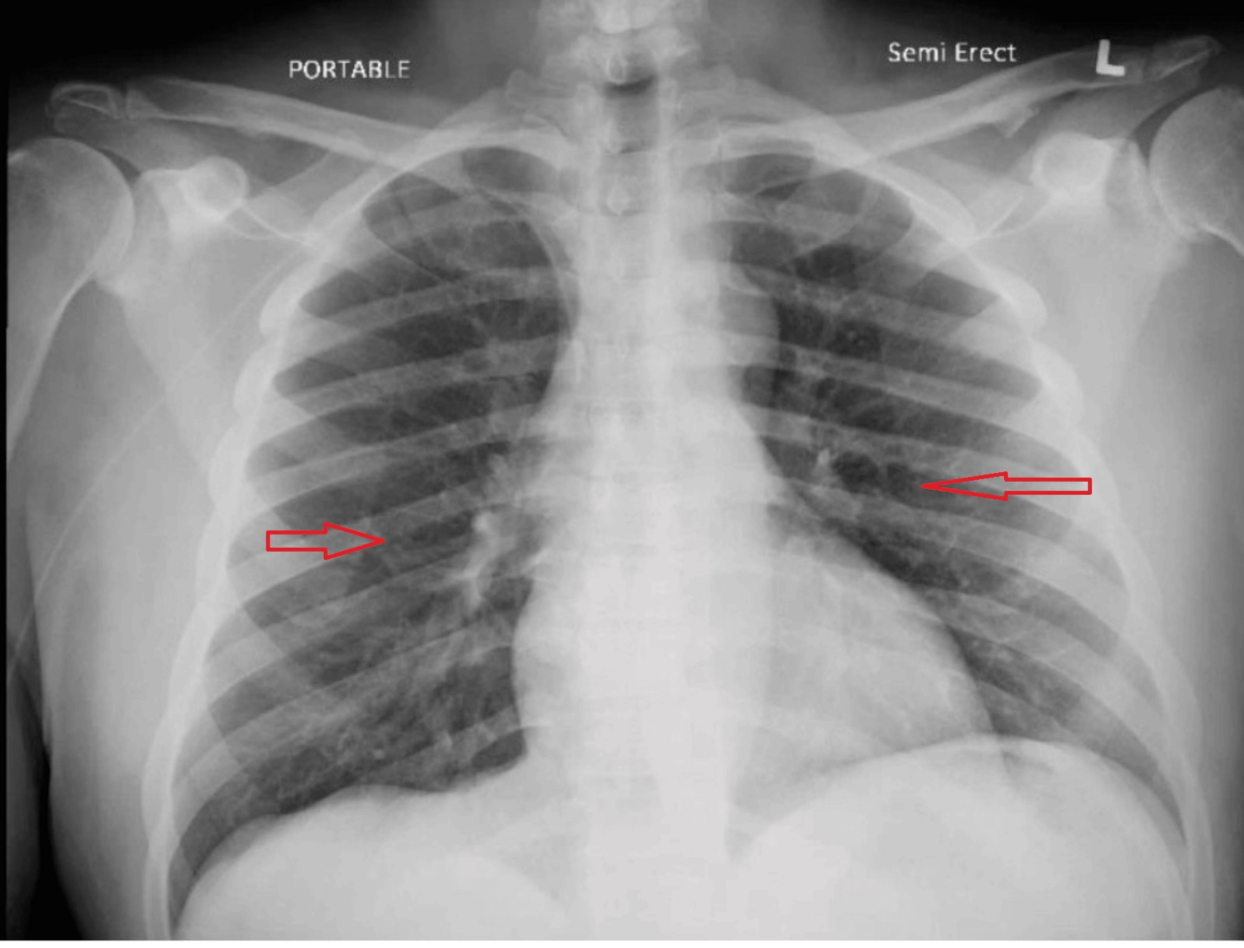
Prior chest X-ray showing no infiltrates or abnormalities.
